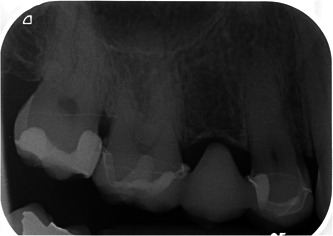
Sample radiograph of tooth‐supported fixed dental prostheses, after a follow‐period of 4 years
